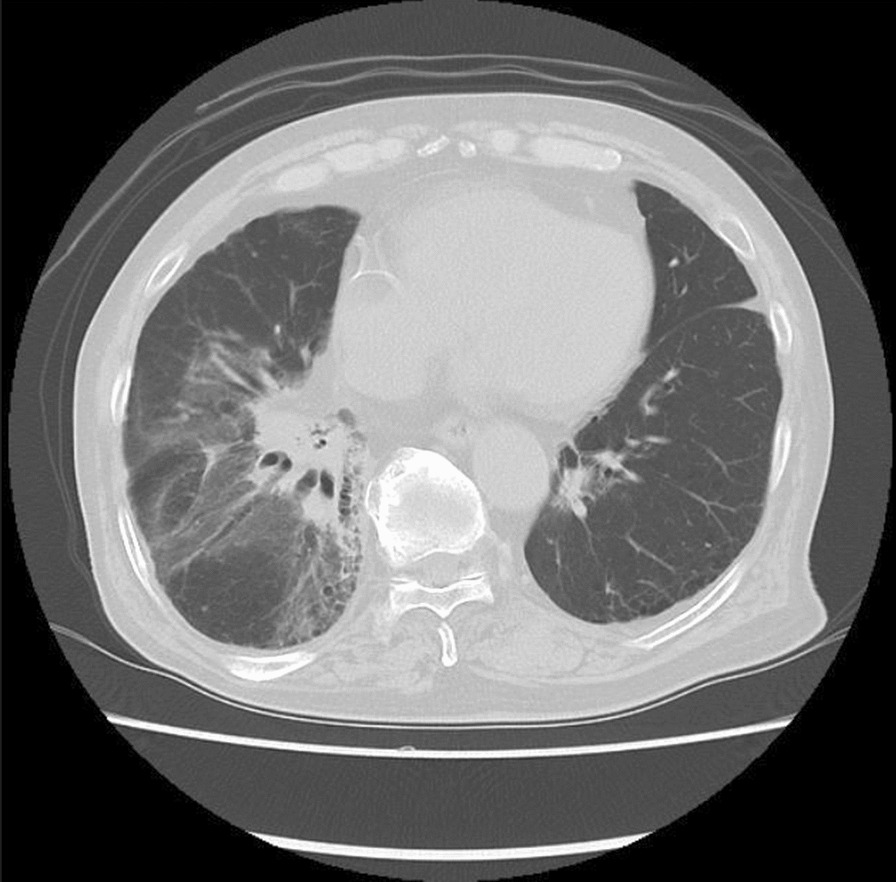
Computed tomography 11 months after the first stereotactic body radiation therapy. Ground-grass appearance consistent with stereotactic body radiation therapy beams was observed
